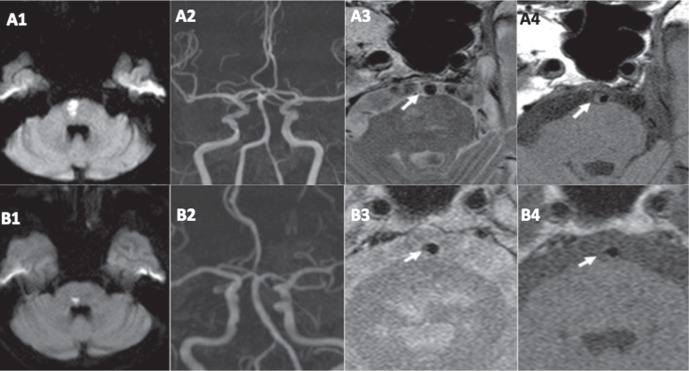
High resolution MR for patient with isolated paramedian pontine infarction (A: paramedian pontine infarction; B: lacunar pontine infarction; 1: diffusion-weighted image; 2: TOF MRA; 3: T_2_-weighted image; 4: T_1_-weighted image). TOF MRA (A2) shows slight narrowing of basilar artery in a patient with paramedian pontine infarction which is seen as atherosclerotic plaque (arrow). TOF MRA (B2) shows normal caliber of basilar artery in a patient with lacunar pontine infarction, whereas atherosclerotic plaque are seen on T_1_- (B3) and T_2_- (B4) weighted MRI (arrow). MRI, magnetic resonance imaging; MRA, magnetic resonance angiography; TOF, time-of-flight.
